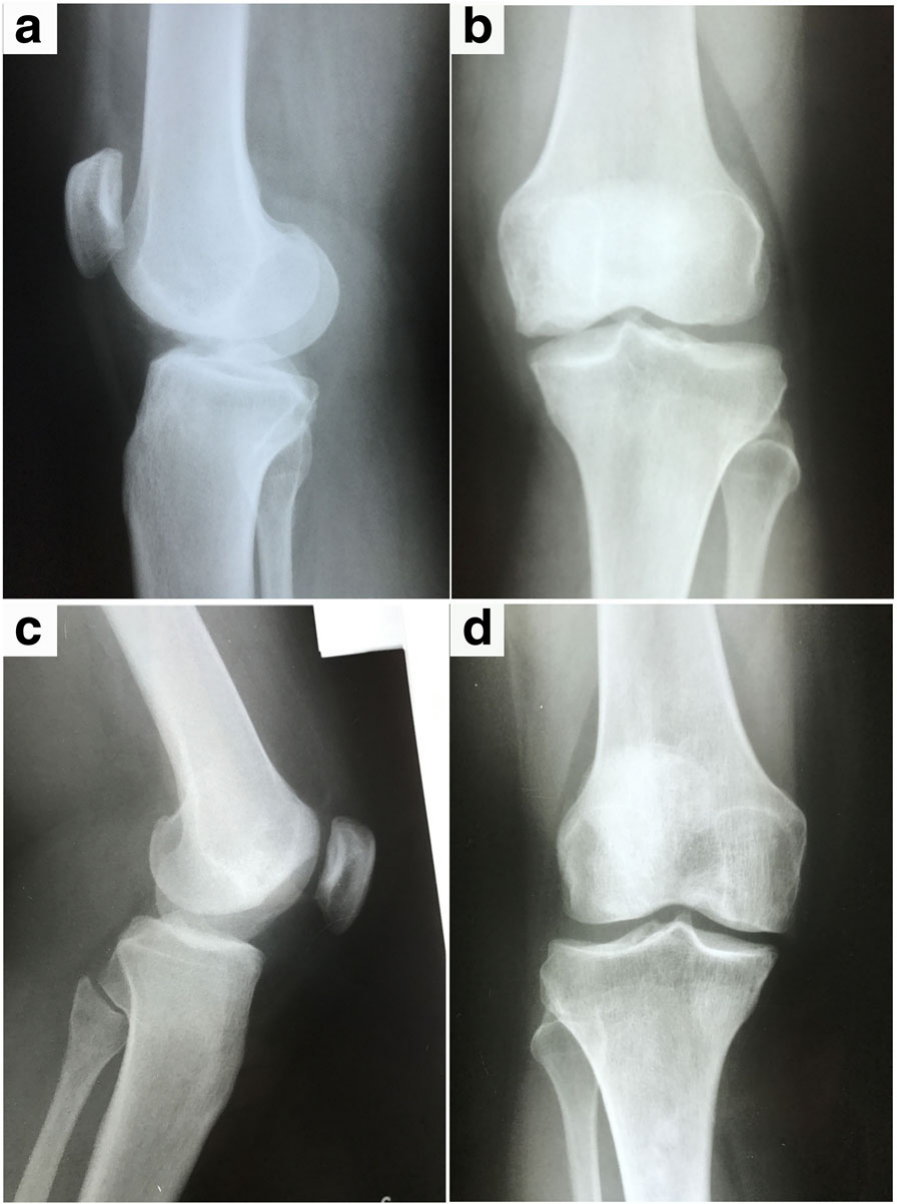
Radiographic imaging after successfully treatment with BMC. a, b Radiographs of the patient displayed on Fig. 1, 36 months postoperatively showing joint preservation and no subchondral bone fracture; (c, d) Radiographs of the patient displayed on Fig. 2, 60 months postoperatively. The joint space was preserved and the patient was asymptomatic ate final follow-up
